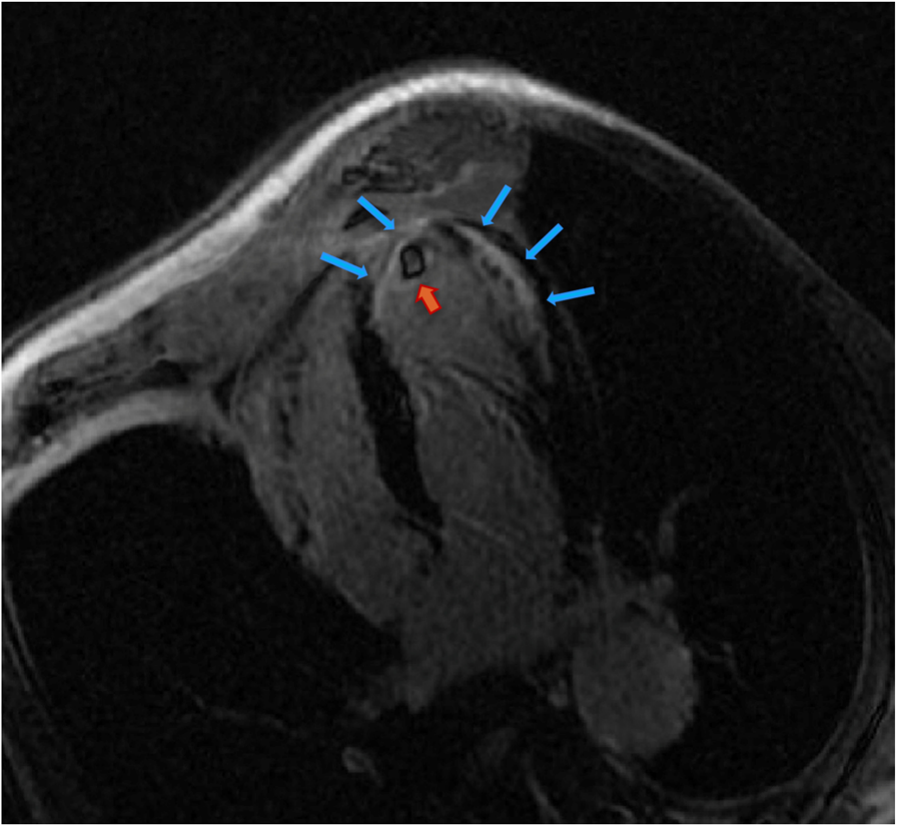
Delayed-enhancement cardiac MR image showing apical intraventricular thrombosis (*orange arrow*) and late gadolinium enhancement (*blue arrows*) in the four-chamber view
